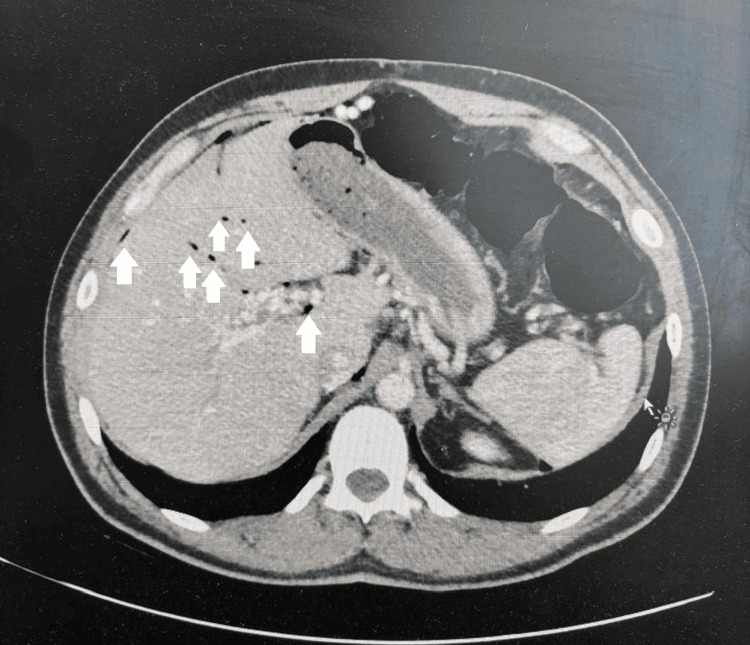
Transverse abdominal CT scan with IV contrast showing benign pneumoperitoneum.
